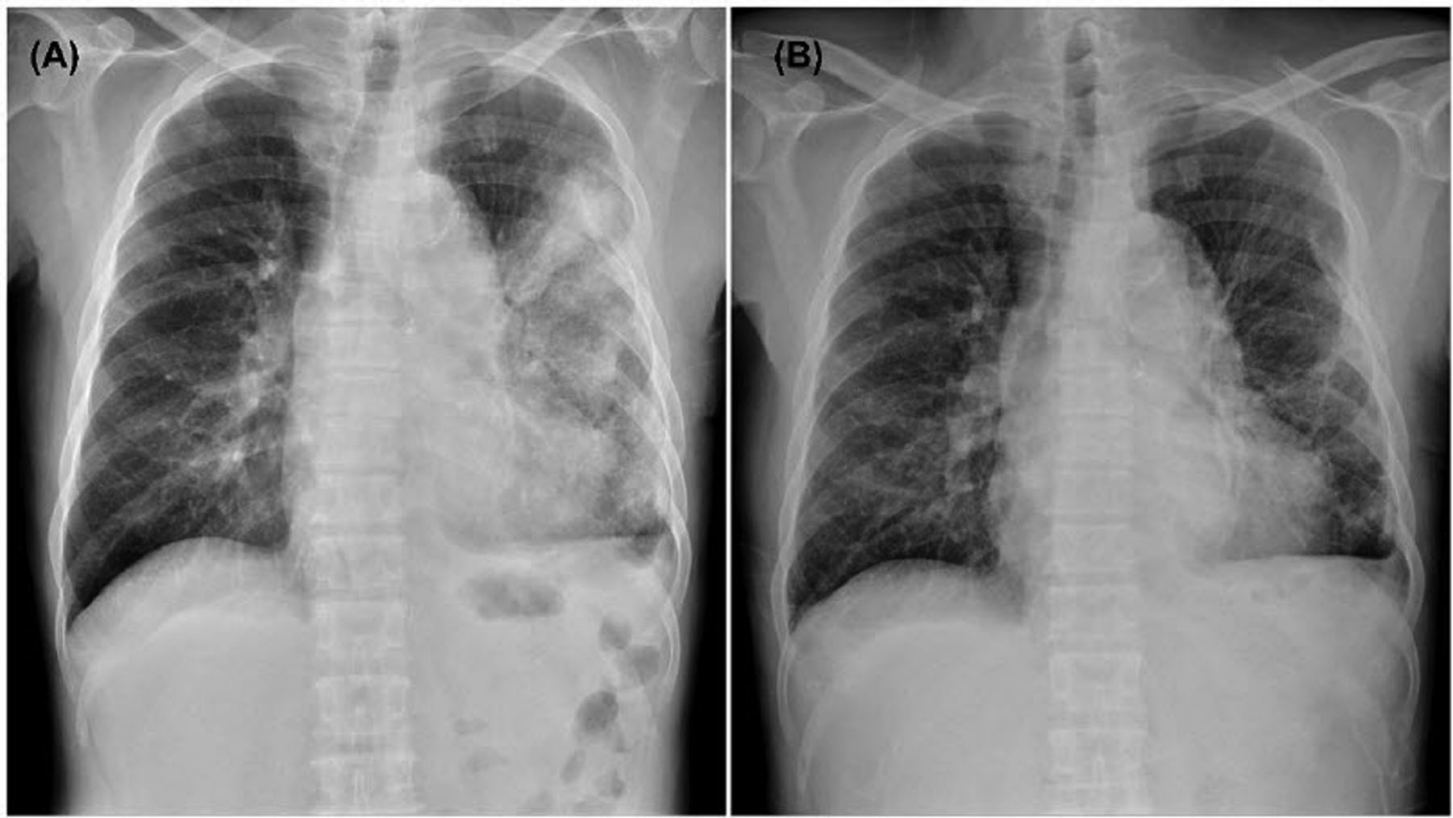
Chest plain films show patchy infiltrates and dense consolidation in the left lung upon admission (A) and apparent resolution of consolidation in the left lung on hospital day 15 (B).
